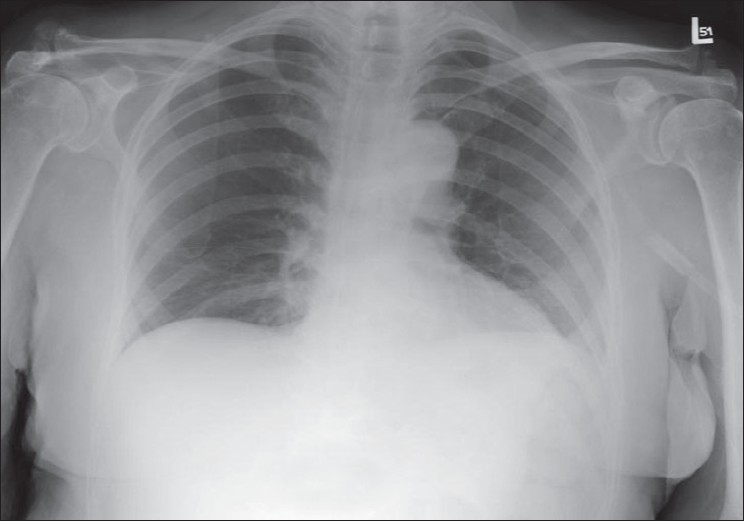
A normal AP chest radiograph of an ICU patient
